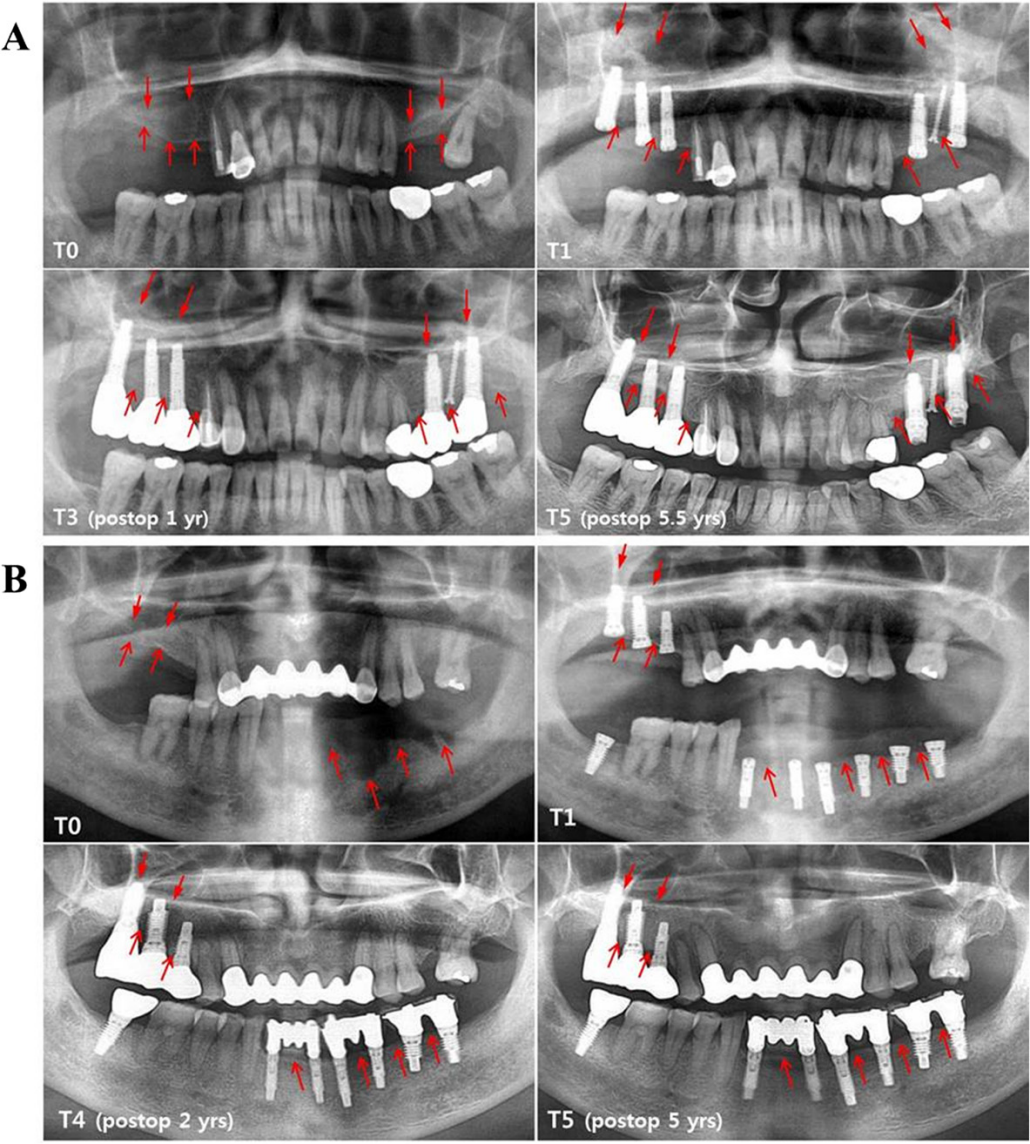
Long-term panoramic evaluation of simultaneous dental implantation cases with autologous iliac bone (a) and intraoral jaw bone grafts (b). a Dental implants are placed in both maxillary posterior ridges with subantral inlay-type iliac block and particulate bone graft. In panoramic analysis, the augmented alveolar bone heights in both maxillary posterior ridges (arrows in T3) are remarkably vertically reduced at the 5.5-year follow-up (arrows in T5). In particular, the radiograph of T5 (5.5 years postoperatively) shows coincidental bone resorption in the marginal alveolar bone (open arrows) and sinus floor (closed arrows) compared with radiographs at T1 or T3, indicating that the long-term grafted bone resorption could be related to the shrinkage volume of grafted iliac bone as well as peri-implantitis. b A case of intraoral jaw bone graft and simultaneous implantation. Implant fixtures are simultaneously placed and jaw bone is grafted onto the sinus floor (subantral inlay-type) and on the exposed fixtures in lower alveolar ridges (onlay-type). The grafted jaw bone is well maintained and shows a lesser vertical bone reductive pattern than the iliac bone graft in the marginal alveolar bone (open arrows) and sinus floor (closed arrows)
